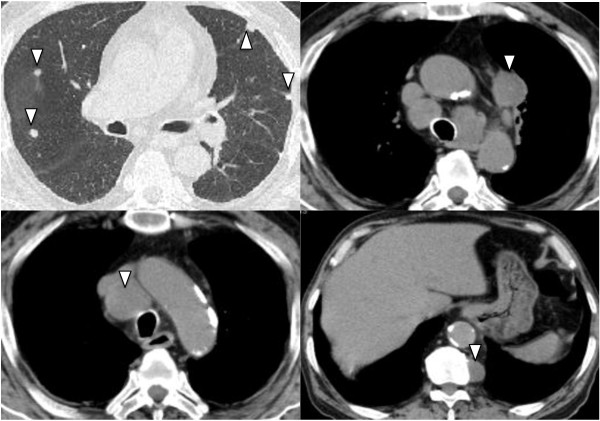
Computed tomography images of disseminated NTM development. The white triangles indicate pulmonary nodules, mediastinal lymph nodes, and paravertebral abscess.
